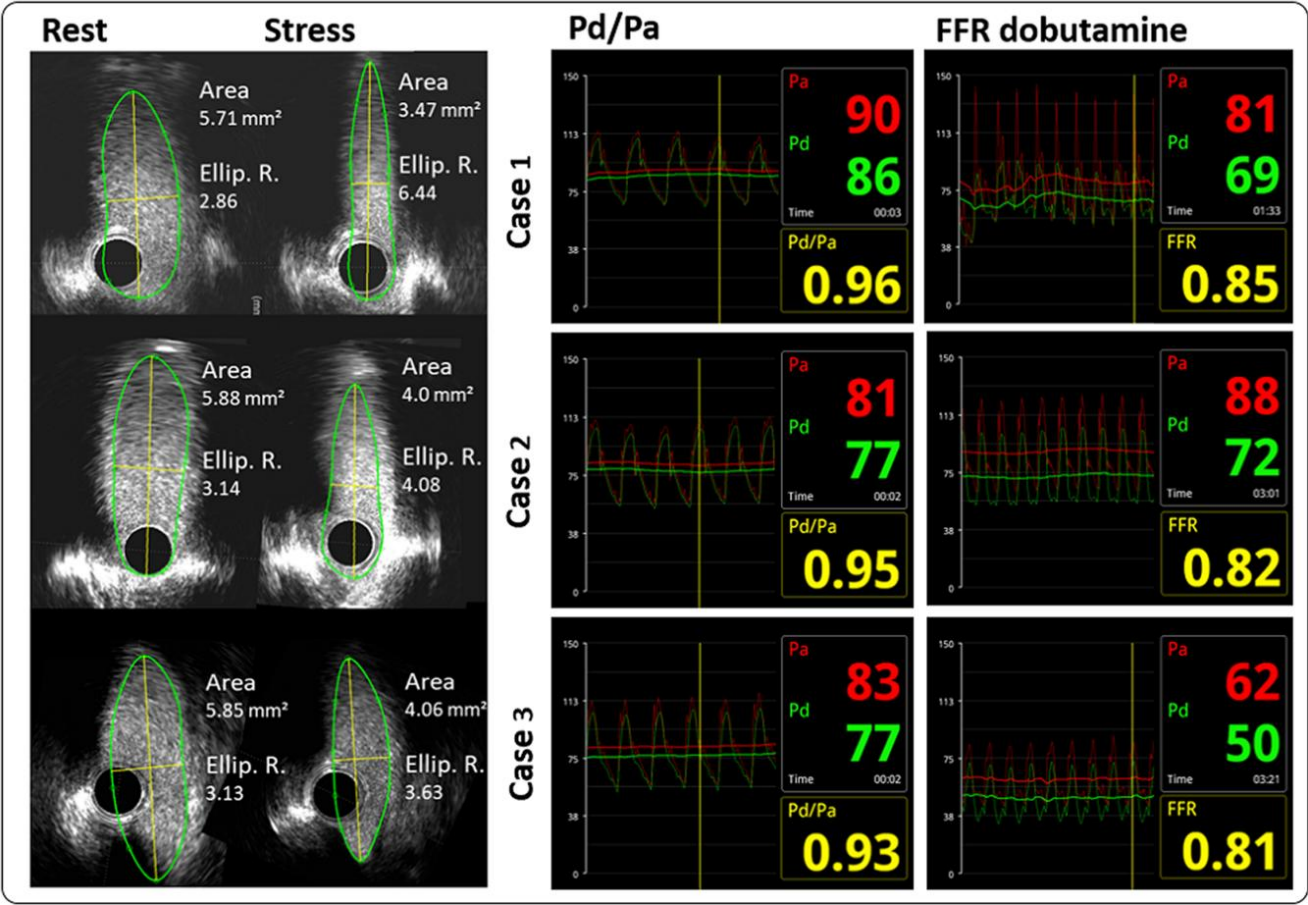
Intravascular ultrasound images of all three cases showing increasing ellipticity and decreasing lumen area under dobutamine stress (left) and fractional flow reserve curves of all three cases showing no sign of a relevant stenosis (right). FFR, fractional flow reserve; IVUS, intravascular ultrasound images; Pd/Pa, minimum distal coronary (Pd) to aortic pressure (Pa) ratio.
